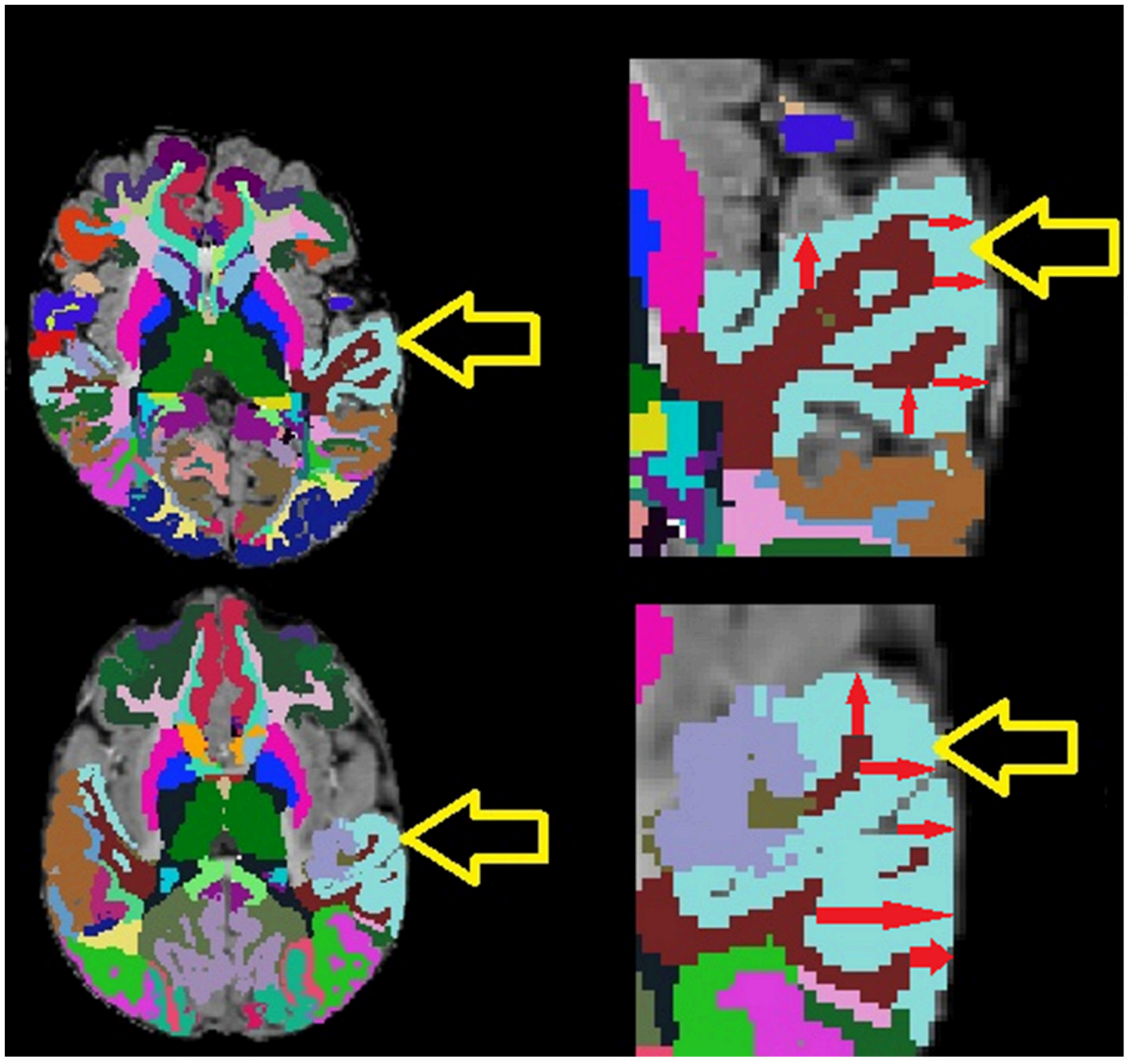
Example MRI examinations with FreeSurfer ROIs (colored regions) with magnifications. Yellow arrows point to the light blue superior temporal cortex, red arrows provide demonstrative examples of some of the many within-region cortical thickness measurements computed by FreeSurfer. Note that the exam on top exhibits lower cortical thickness variability than the exam on the bottom which exhibits highly varying cortical thickness measurements.
